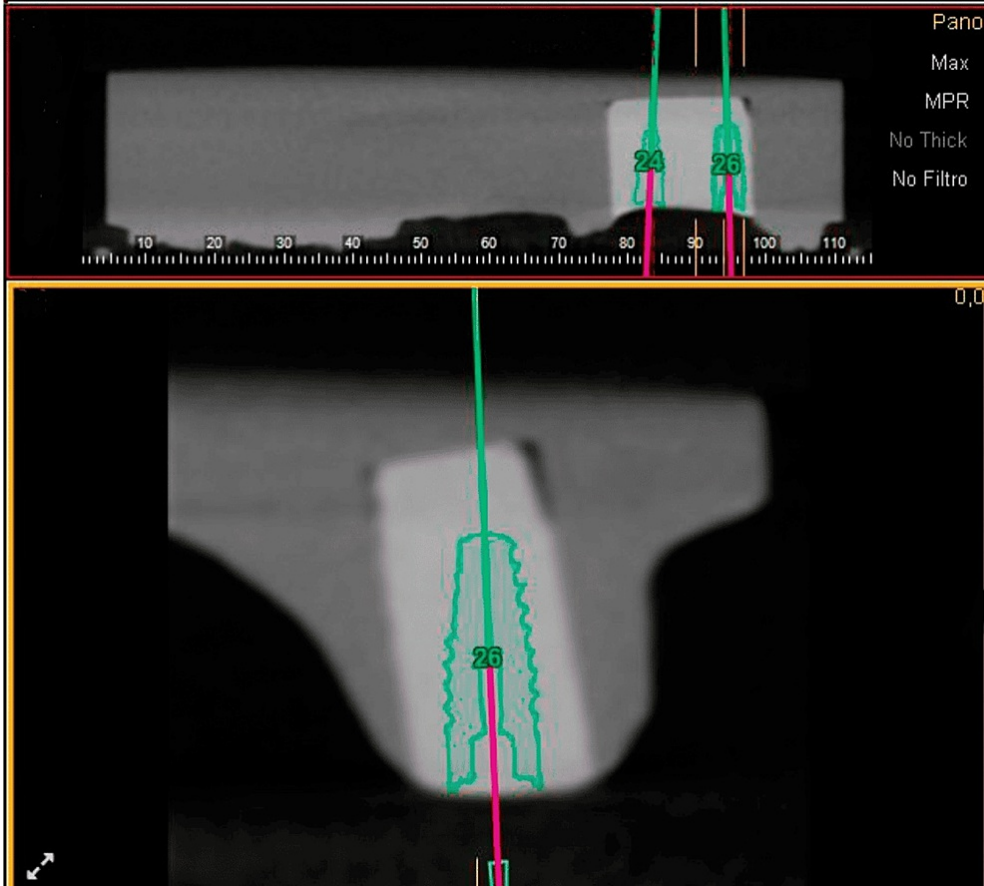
Scan of the Cast Done by CBCT and Then Virtual Planning for the Implant Placement CBCT: cone-beam computed tomography
